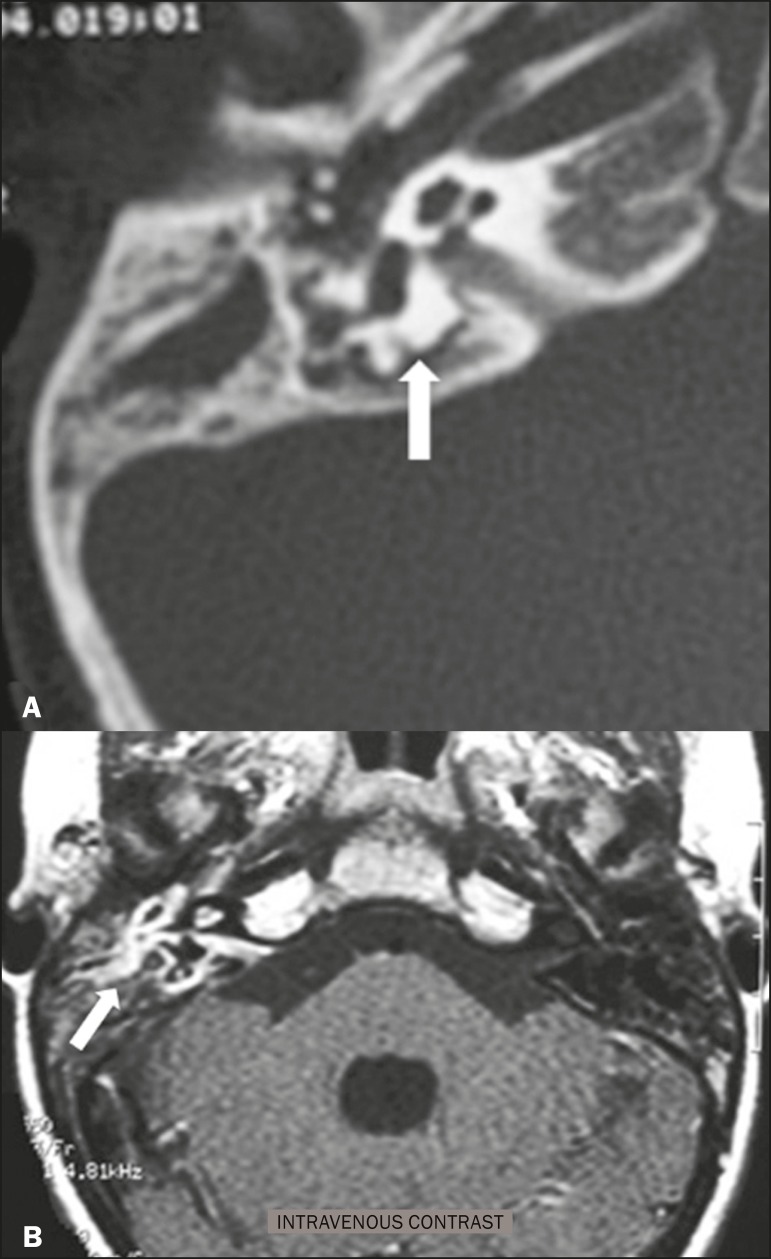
Otomastoiditis complicated by osteomyelitis. A: Axial CT
